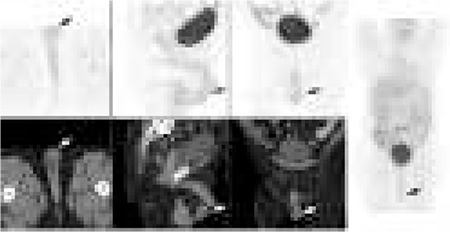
Complete resolution of the previously increased FDG avid left testis and the left inferior scrotal sac (arrow)
